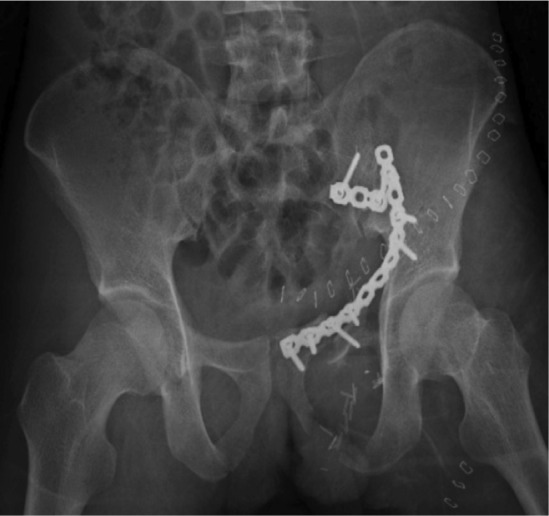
Pelvic radiograph following open reduction and ring and sacroilliac joint internal fixation.
